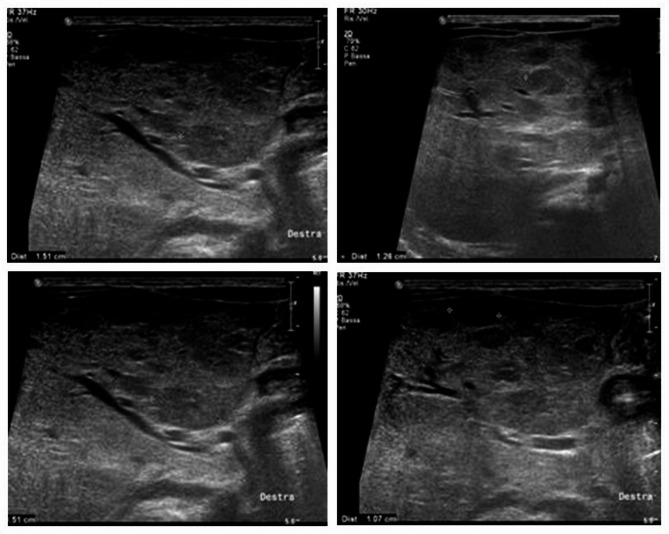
Abdominal ultrasonography at diagnosis showing slight hepatomegaly with well-delineated uncountable hypo-isoechoic lesions with a mean diameter of 15 mm
